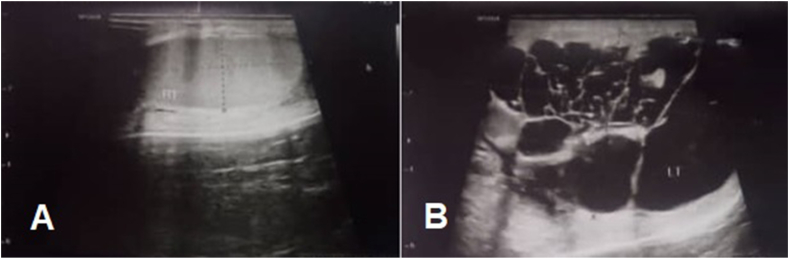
Ultrasound images of the patient. Right Testis (A) and Left Testis (B).
